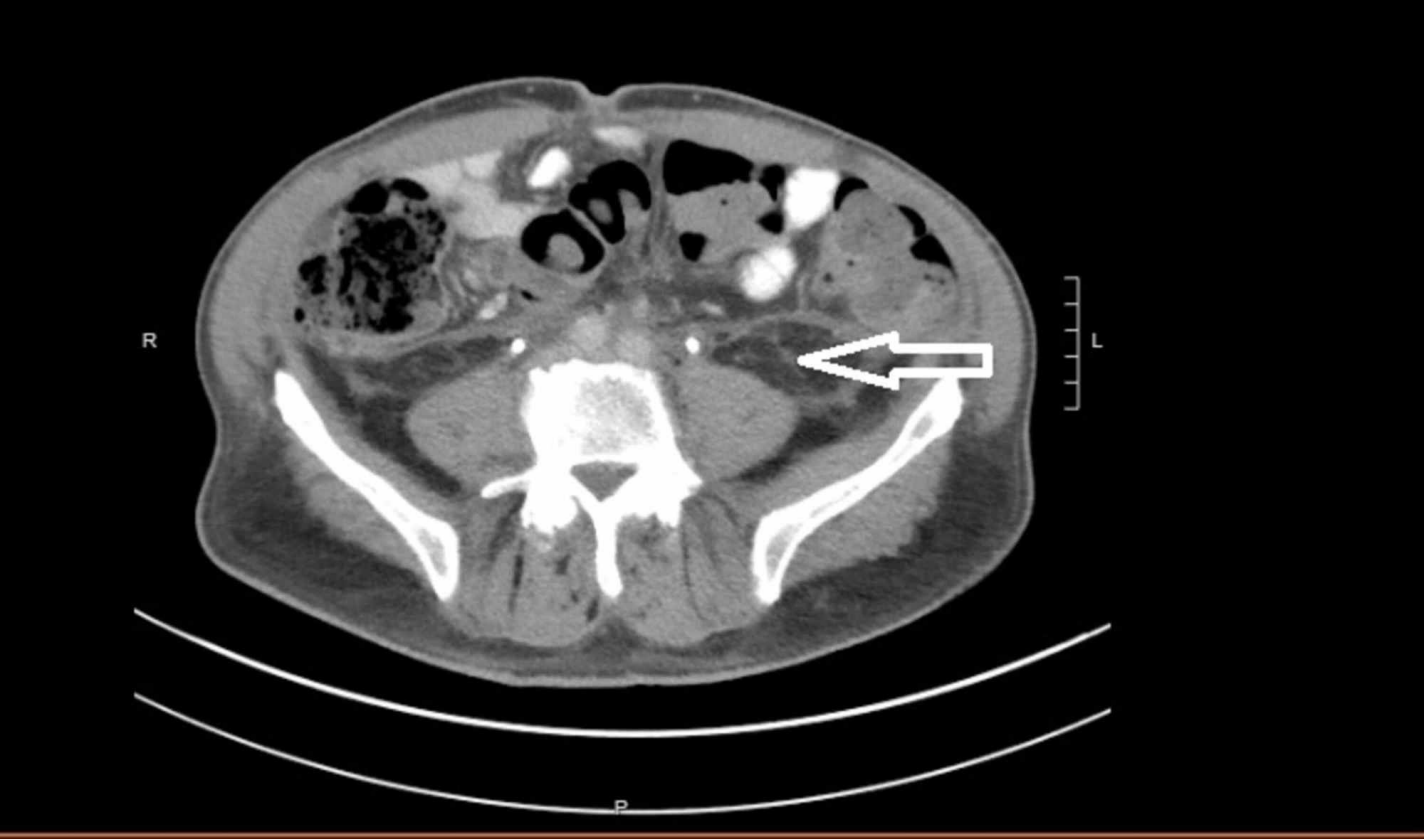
CT shows near resolution of retroperitoneal fibrosis depicted with the arrow.
